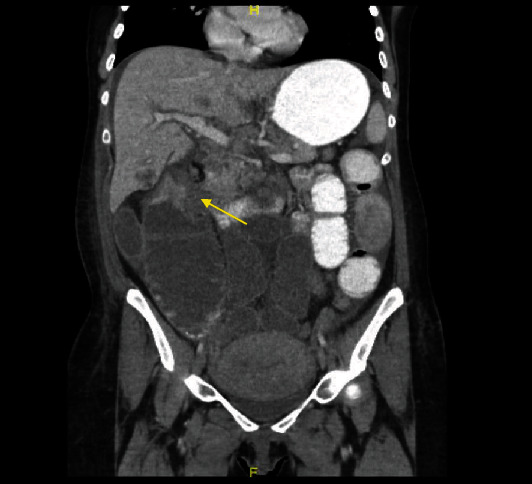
Computed tomography scan showing a mass in hepatic flexure of the colon.
